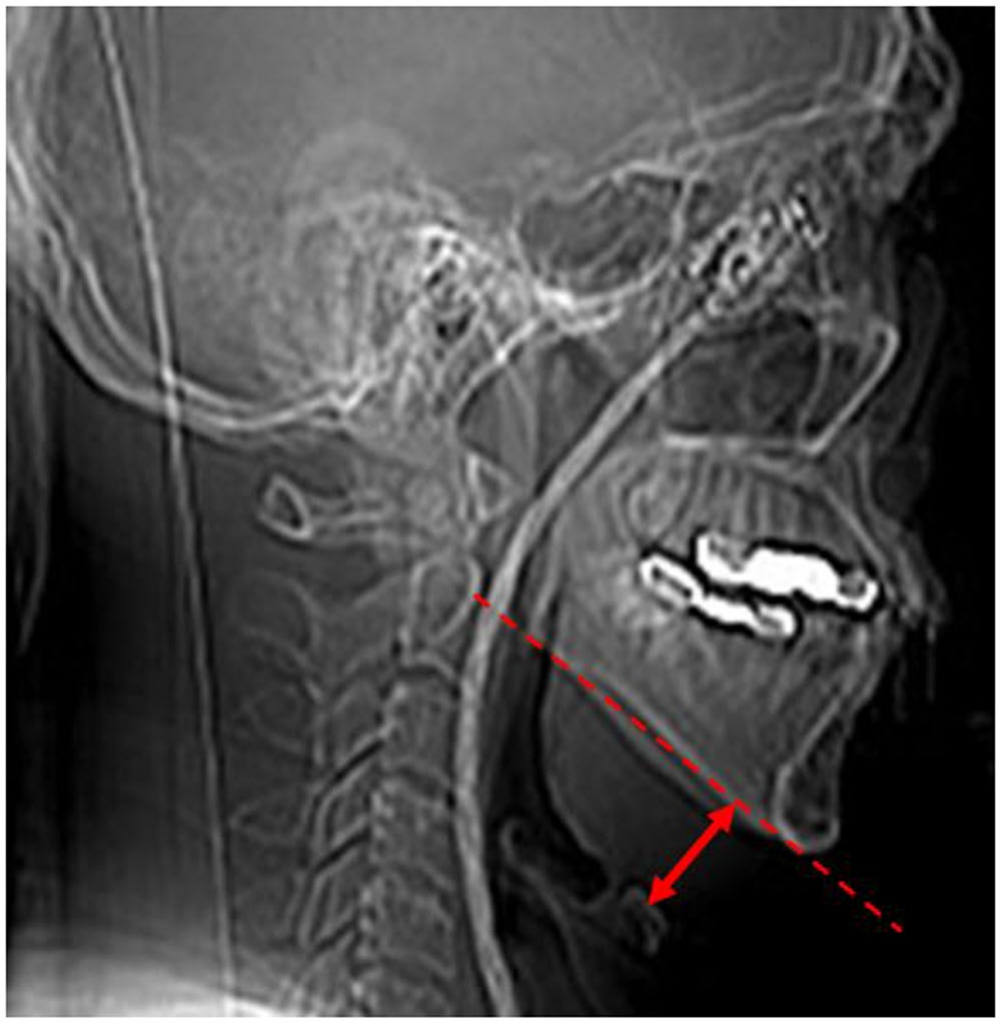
The mandible plane-hyoid distance (MPH) measurement. The MPH (red double arrow) was defined as the vertical distance from mandibular plane (red dotted line) to hyoid bone using a plain film of a computed tomography scan.
